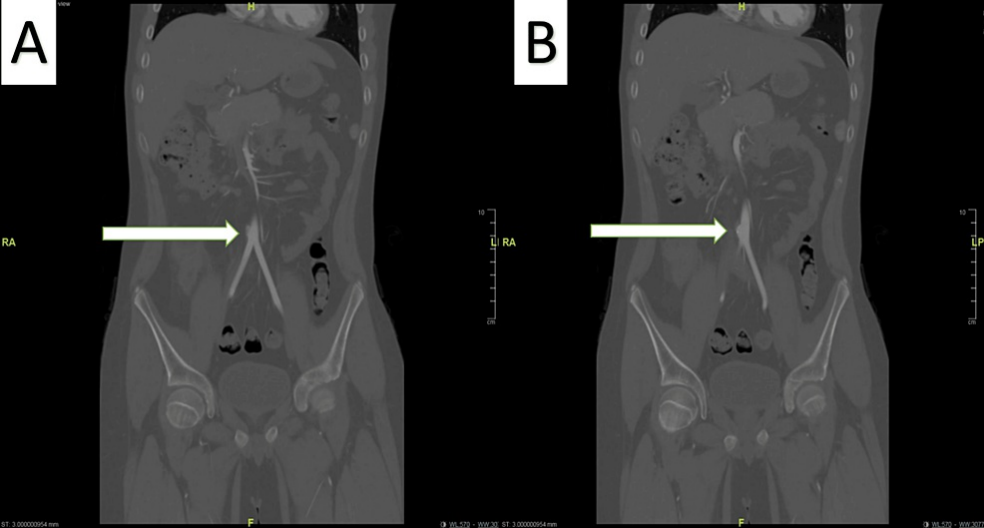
Postoperative 5-Month Follow-Up Magnetic Resonance Imaging Scans A: Previously noted aneurysm of the lower abdominal aorta above the bifurcation is no longer demonstrated (white arrow); B: No abdominal aortic luminal or perivascular abnormality is demonstrated (white arrow).
